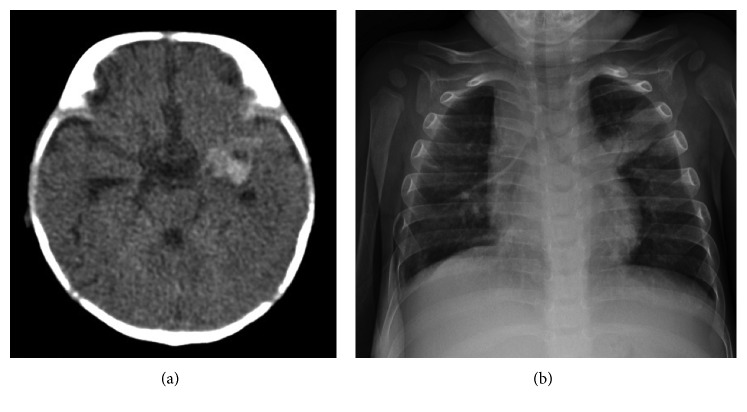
(a) CT brain showed hyperdensity lesion size of 1.5 × 1.8 cm at the left temporal lobe. (b) Chest X-ray revealed patchy infiltration at the left upper lobe.
